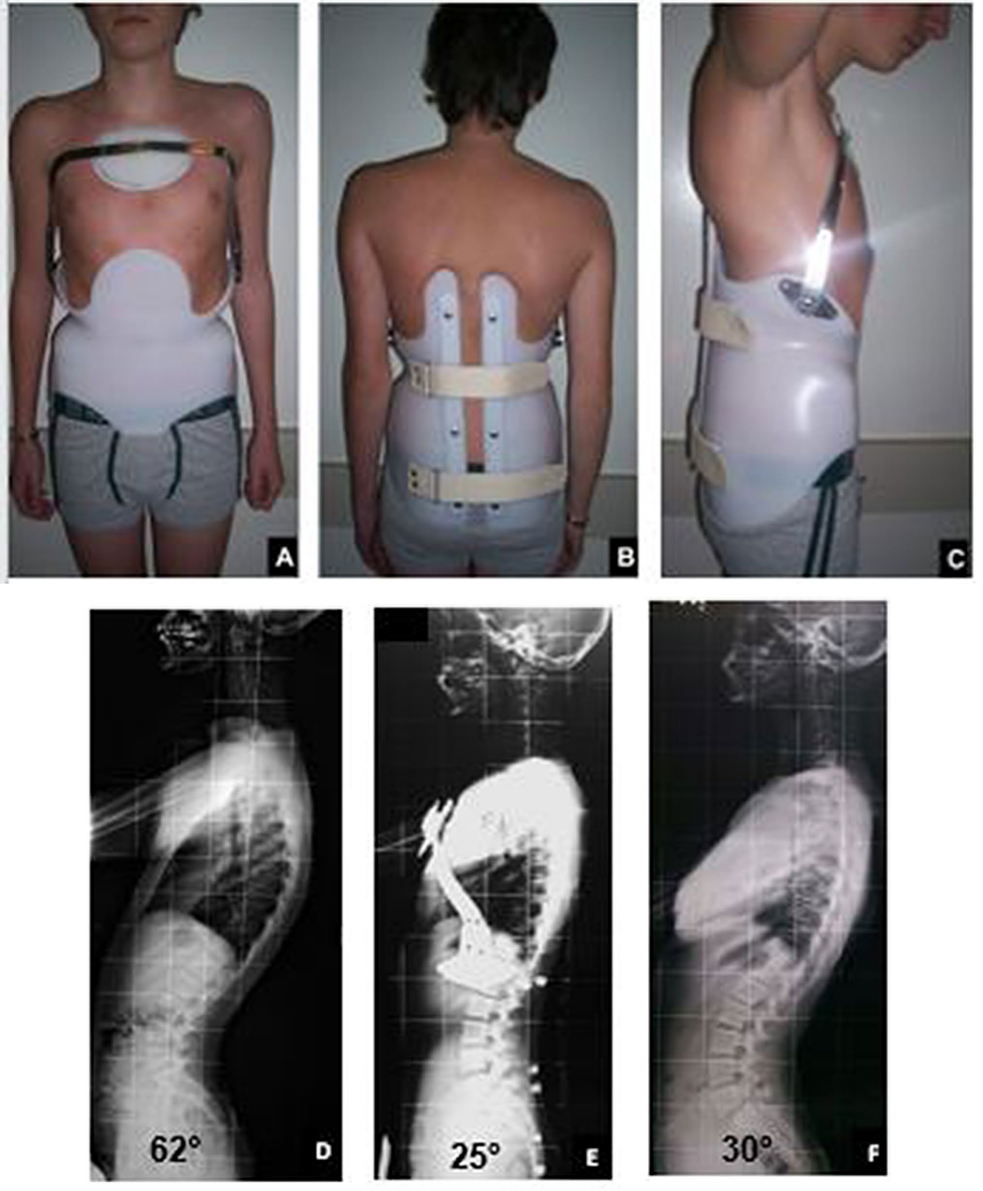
A 14-years old patient managed with MA brace. a Anterior view. b Posterior view. c Lateral view. d Lateral full spine X-rays at recruitment (*T*_0_). e Lateral full spine in-brace X-rays (*T*_1_). f Lateral full spine X-rays at the end of treatment (*T*_3_)
